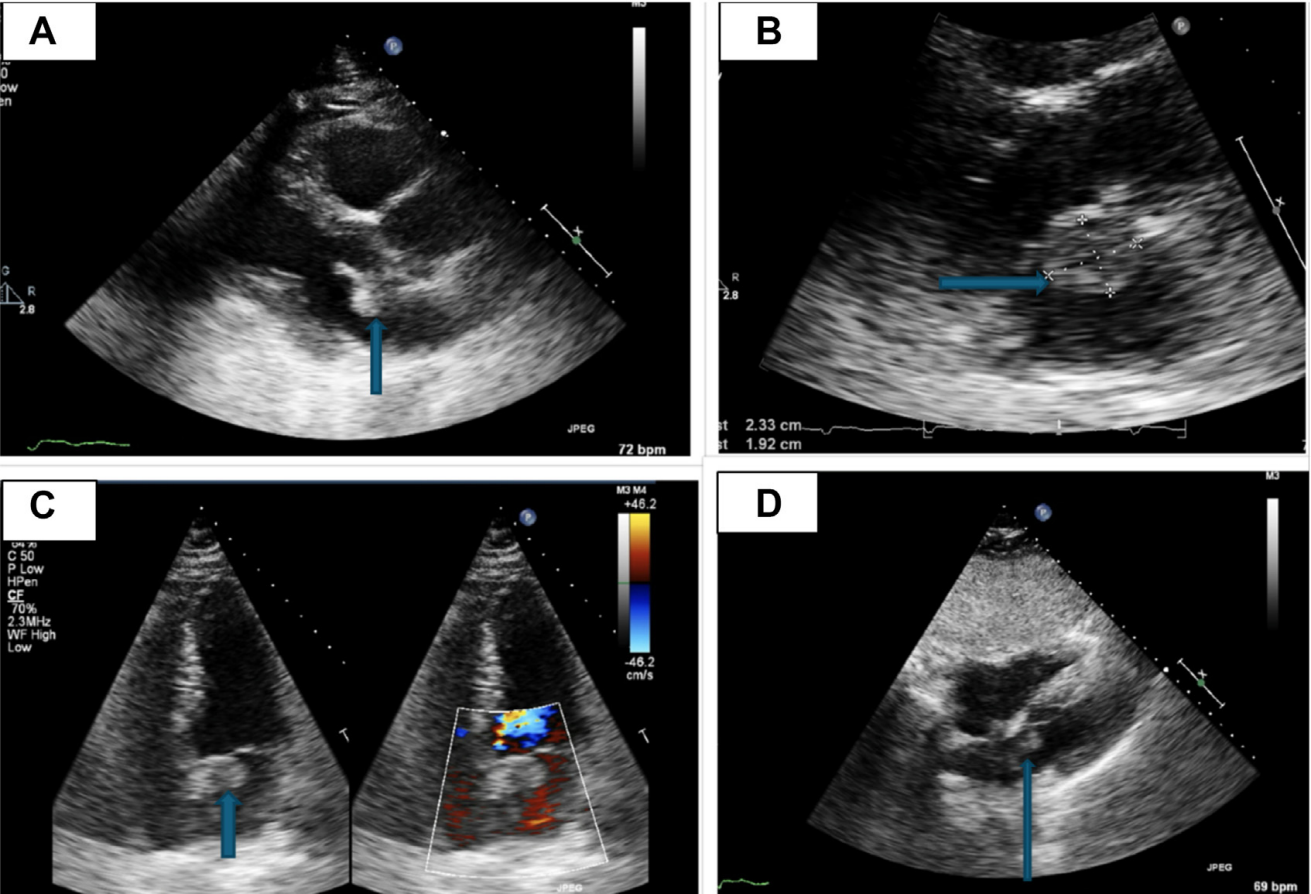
Transthoracic Echocardiogram Transthoracic echocardiogram (TTE) reveals a 2.3 × 1.9 cm mobile mass in the left atrium (A, B), indicated by blue arrows. The mass appears attached to the interatrial septum, as shown by arrows in C and D, consistent with an atrial myxoma.
